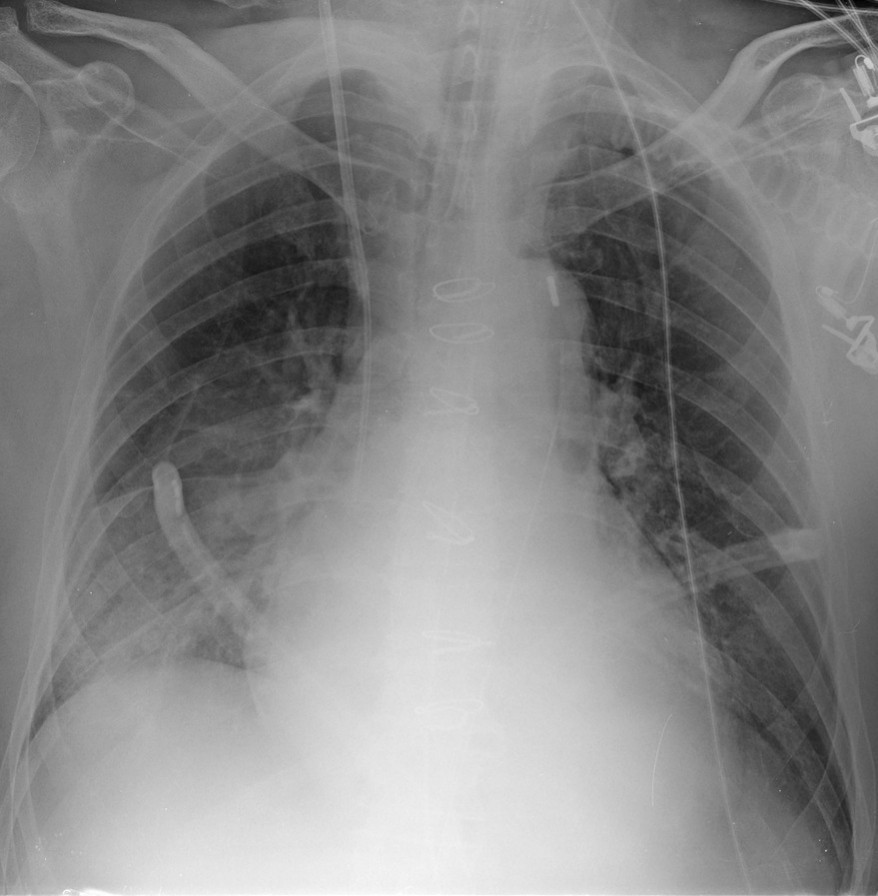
Chest radiograph performed in the recovery room showing a right basal pleural effusion.
